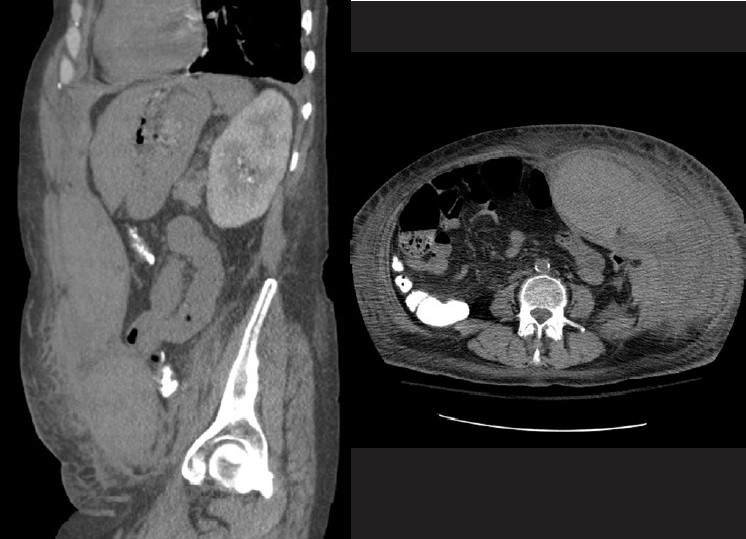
CT Scan images of abdominal wall hematoma (H)
